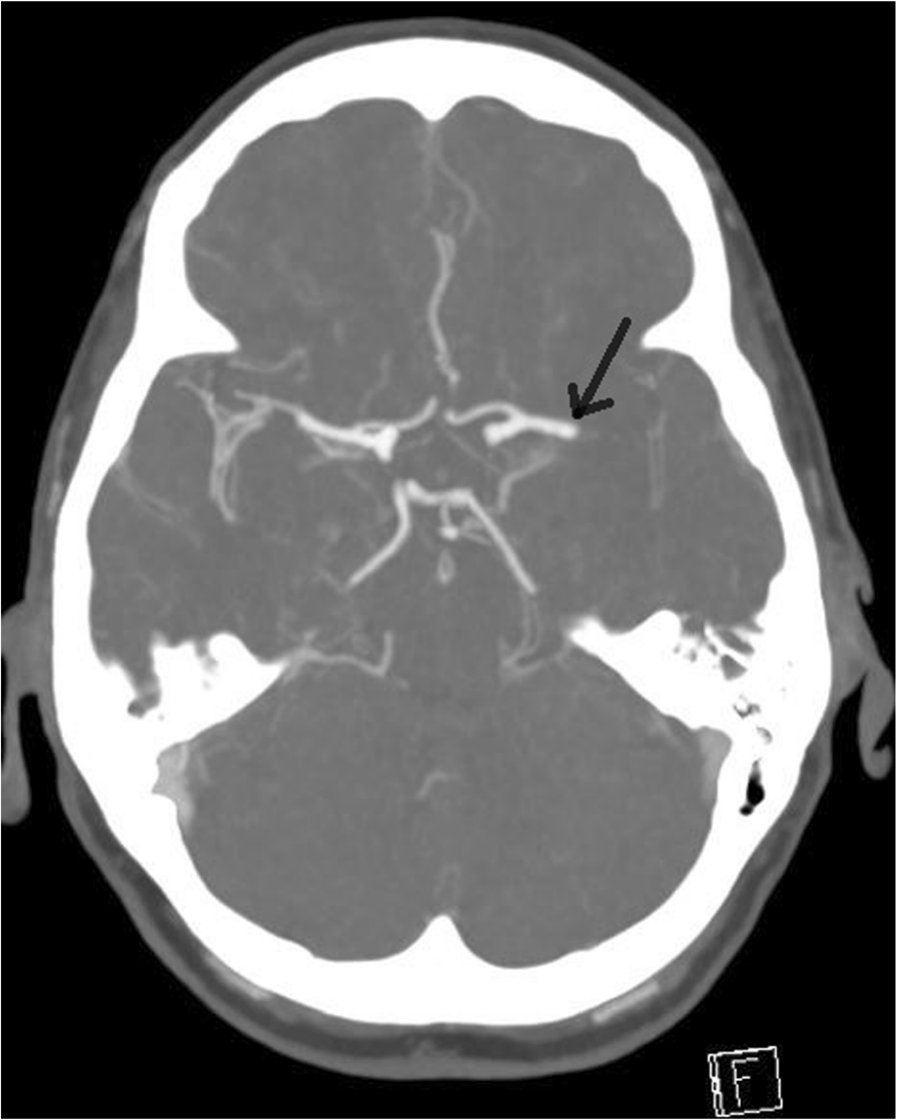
CTA done during the stroke call, demonstrating filling defect in the left proximal MCA (arrowed)
